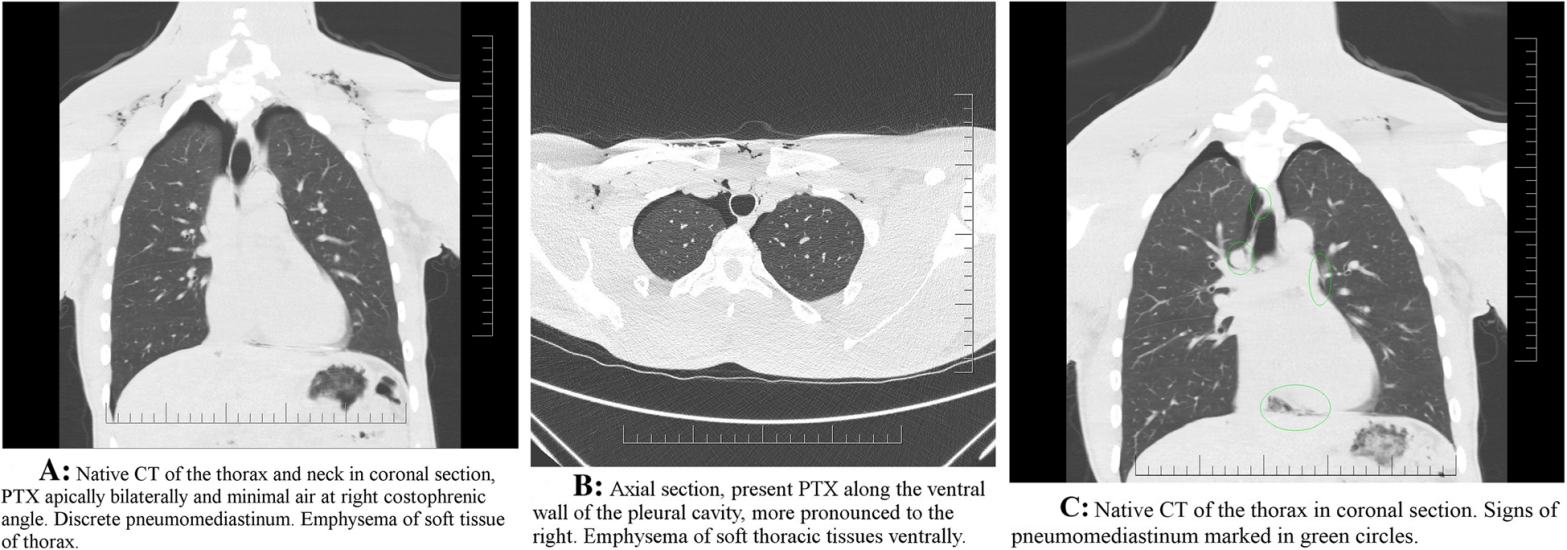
a Native CT of the thorax and neck in coronal section, PTX apically bilaterally and minimal air at right costophrenic angle. Discrete pneumomediastinum. Emphysema of soft tissue of thorax. b Axial section, present PTX along the ventral wall of the pleural cavity, more pronounced to the right. Emphysema of soft thoracic tissues ventrally. c Native CT of the thorax in coronal section. Signs of pneumomediastinum marked in green circles
